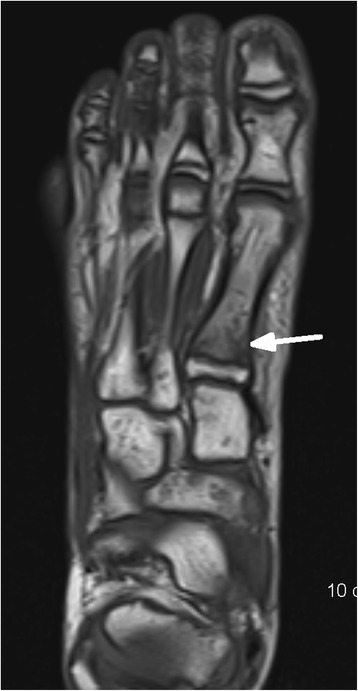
Axial T1-weighted TSE imaging shows a fracture (*arrow*) on the base of the first metatarsal
